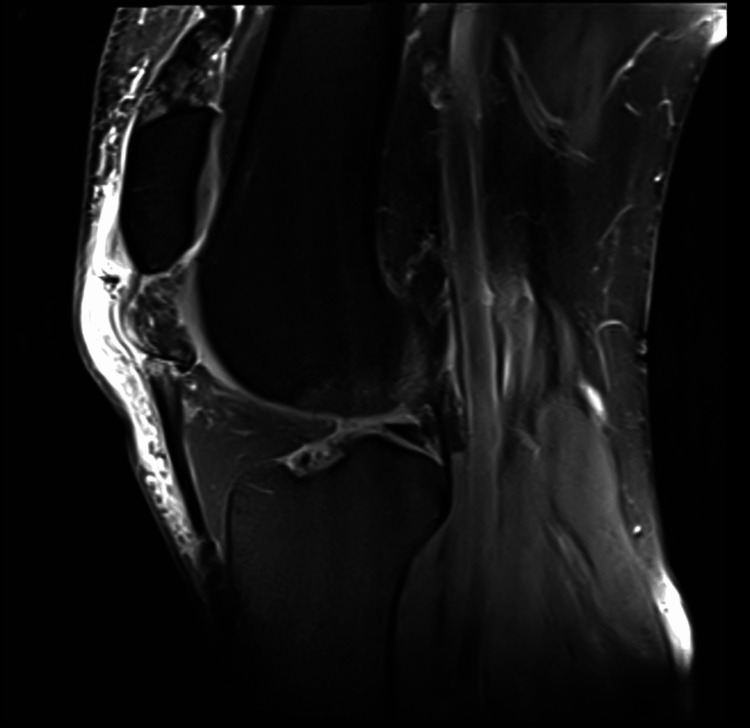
Sagittal MRI demonstrating midsubstance patellar tendon rupture. Sagittal T2-weighted fat-suppressed magnetic resonance imaging (MRI) of the right knee demonstrating a complete midsubstance rupture of the patellar tendon, with proximal displacement of the patella and surrounding soft tissue edema.
